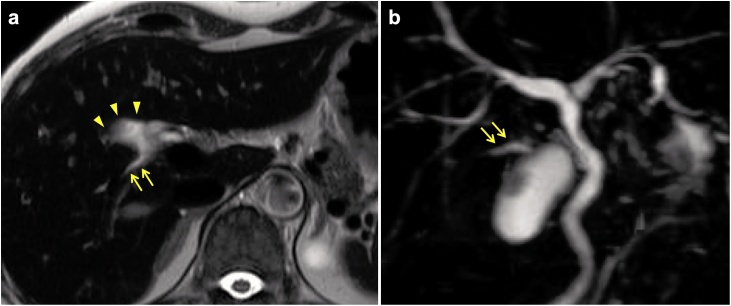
Magnetic resonance image (a) and magnetic resonance cholangiopancreatography (b) showed an aberrant bile duct (arrows) in the gallbladder fossa. Gallbladder was indicated by arrow heads.
